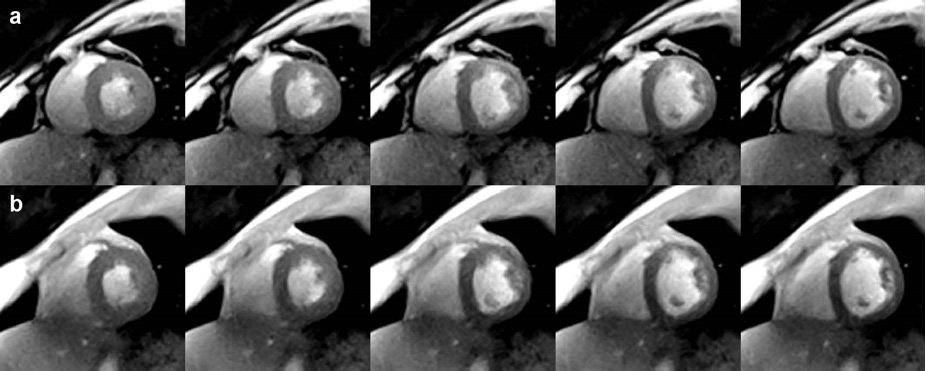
Simultaneous opposed-phase and in-phase real-time radial FLASH CMR. T1-weighted images (spoiled multi-echo FLASH, TR = 3.1 ms, flip angle 8°, 11 spokes) of a short-axis view with (a) an opposed-phase (TE = 1.3 ms) and (b) in-phase condition (TE = 2.4 ms) for overlapping water and fat signals (2.0 mm resolution, 8 mm section thickness, 34 ms total acquisition time). The images cover a post-systolic expansion phase by every second frame (68 ms apart). They were selected from respective real-time CMR movies reconstructed by nonlinear inversion and zoomed by a factor of 1.5.
